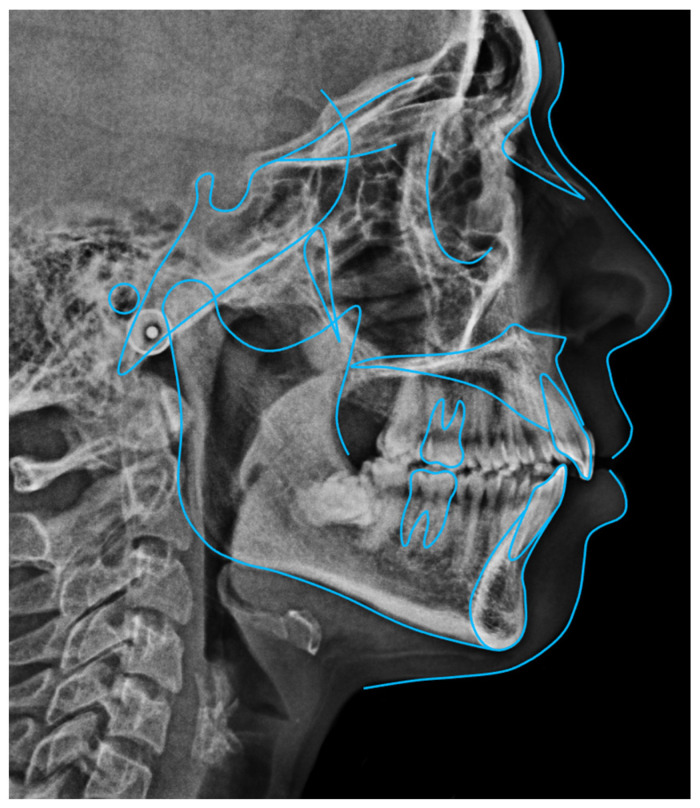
Initial cephalometric tracing of Clinical Case 2. The blue lines represent the cephalometric tracing overlaid on the radiographic image.
